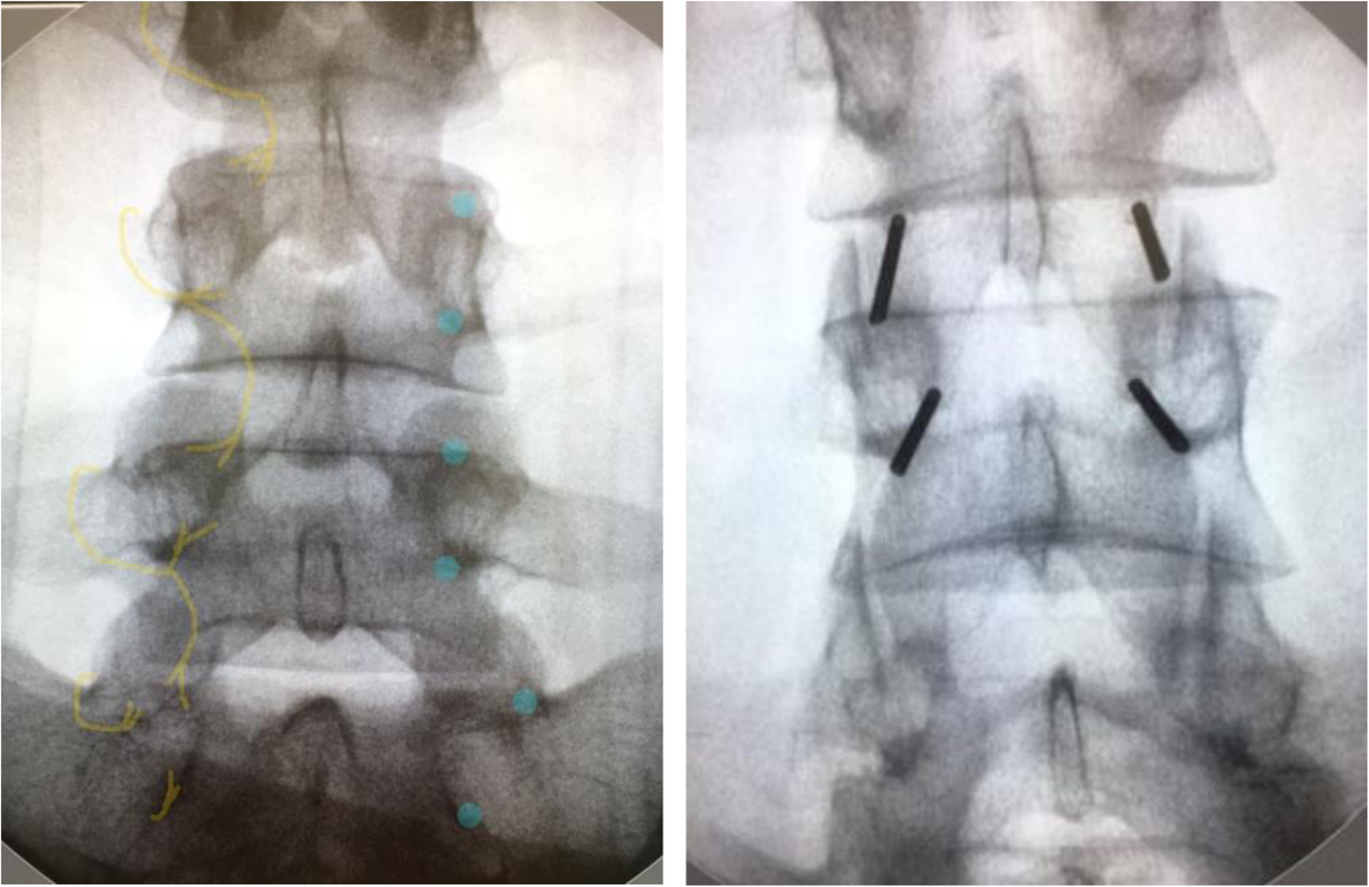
X-ray (anterior posterior view) on the right shows the cannulaplacements when performing intervention on the lumbar spine. The x-ray on theleft shows the medial branch nerves marked with yellow and the cannulaplacement marked with blue dots
